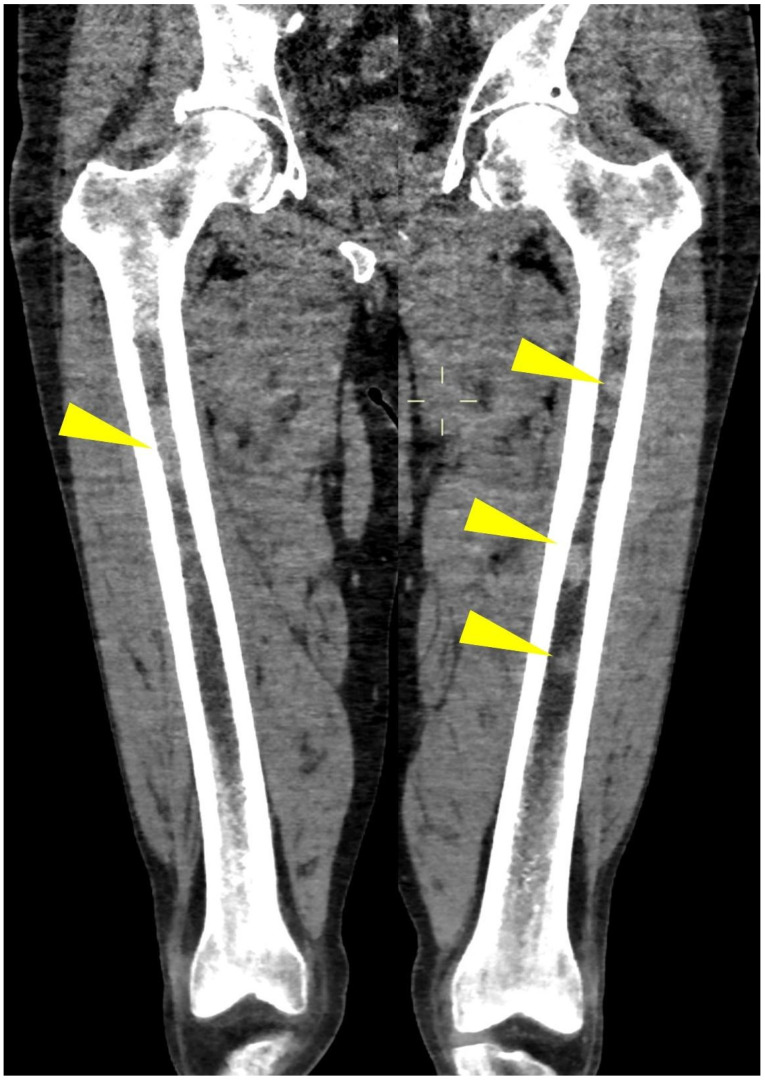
Coronal CT image of (yellow arrowheads) bilateral femoral focal intra-medullary high CT density lesions in a patient with MM.
